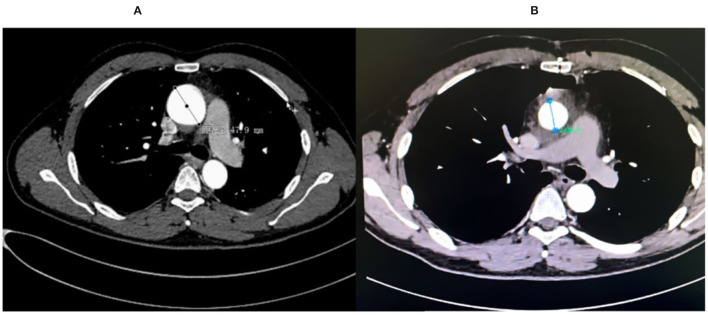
(A) The pre-op CT angiography (CTA) showed ascending aortic aneurysm of 47.9 mm. (B) The post-op CTA scan showed normal ascending aortic size.
